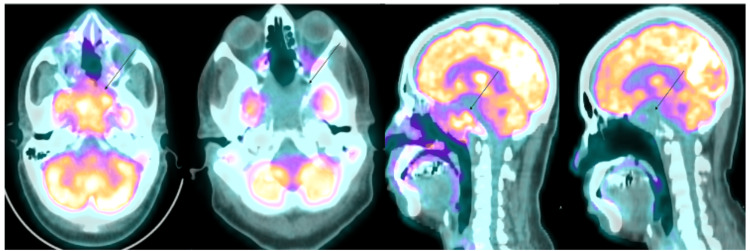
Positron emission tomography (PET) scan Right skull base with standardized uptake value (SUV) of 6.9 before treatment and significantly decreased activity after treatment with radiation and systemic therapy.
